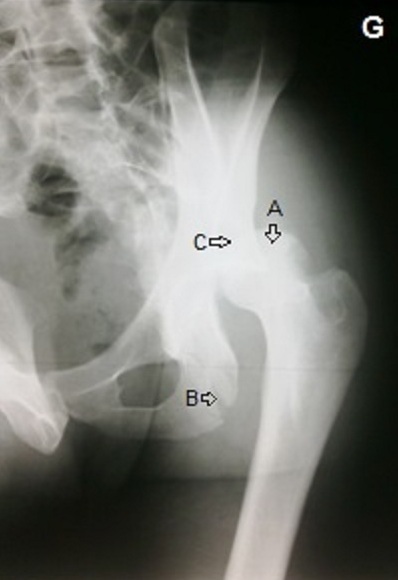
(Patient 1) radiographie de la hanche gauche en ¾ montrant une luxation postérieur de la tête fémoral (flèche A), une fracture de la colonne postérieure (flèche B) avec fracture de la paroi postérieure (flèche C) du cotyle gauche
